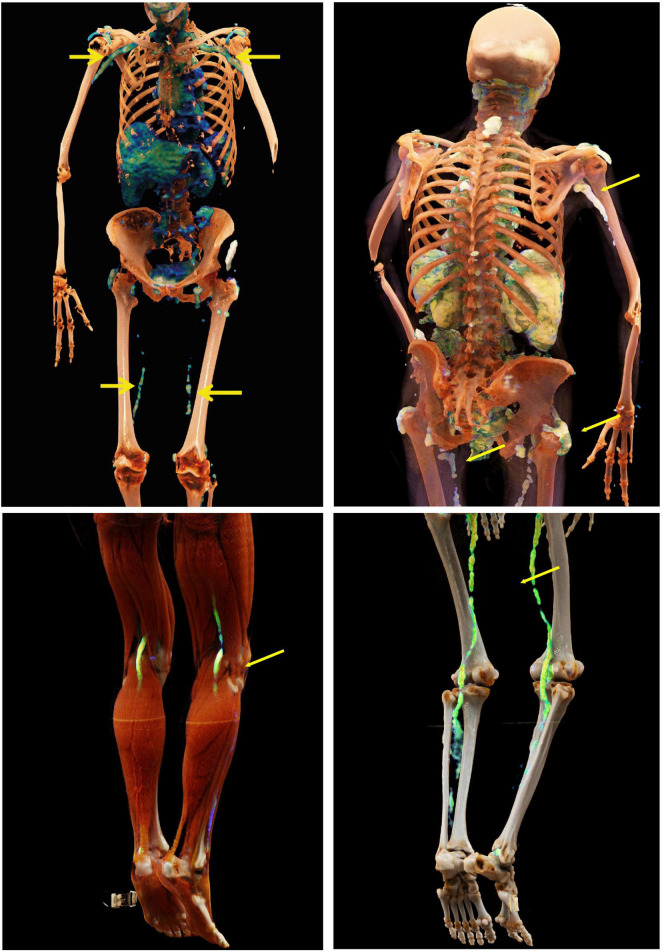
Cinematic rendering of the large vessels in giant cell arteritis. Cinematic rendering based on fusion images from PET and CT showing inflamed vessels (yellow arrows) in giant cell arteritis. Furthermore, inflammation of subacromial bursae is seen, which is associated with polymyalgia rheumatica. By applying CR on fusion images such as PET-CT scans, patients are easily able to understand the origin of inflammatory processes which are happening on vessel level in their body and thus understand why treatment is necessary.
